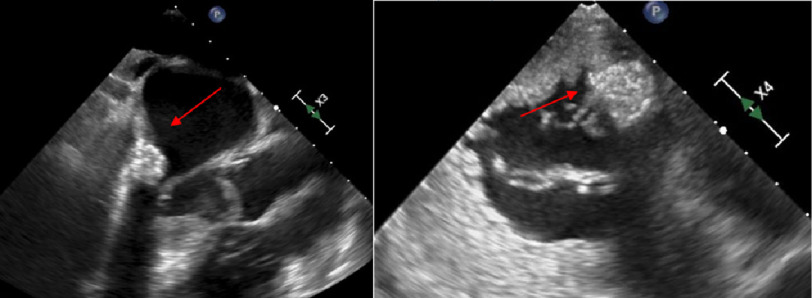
Transesophageal echocardiography imaging (left: mid-esophageal long-axis view, right: transgastric basal short axis view). Red arrows point to the circular echogenic mass, measuring 1.5 by 1.4 cm, near or on the posterior annulus of the mitral valve. There was prolapse of the P3 posterior mitral valve leaflet and severe mitral regurgitation with eccentric jets anteriorly and centrally.
